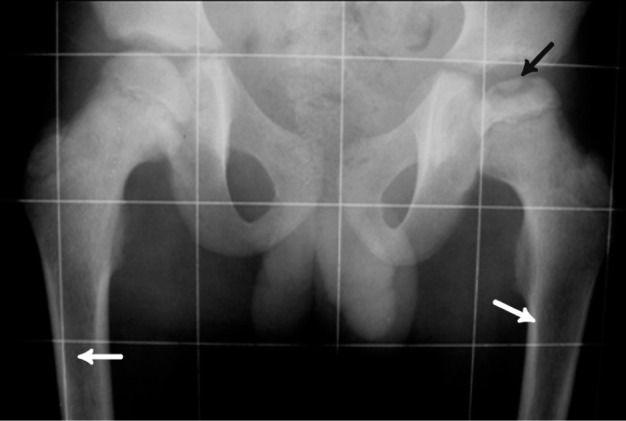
A 9-year-old boy with ALL presenting with groin pain and limping Pelvic X-Ray of this case reveals reduced height of femoral epiphysis with osteochondral fracture on the left side due to avascular necrosis (black arrow). Permeative lesion in both femoral proximal metaphyses are noted (white arrows).
